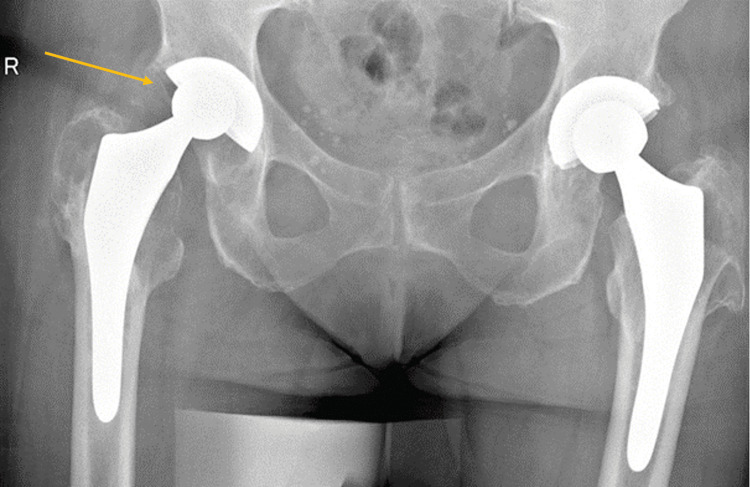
Anteroposterior pelvic X-ray of an 84-year-old patient with polyethylene wear 18 years after right primary total hip arthroplasty performed through the direct lateral approach. Bone scintigraphy revealed loosening of the femoral component.
